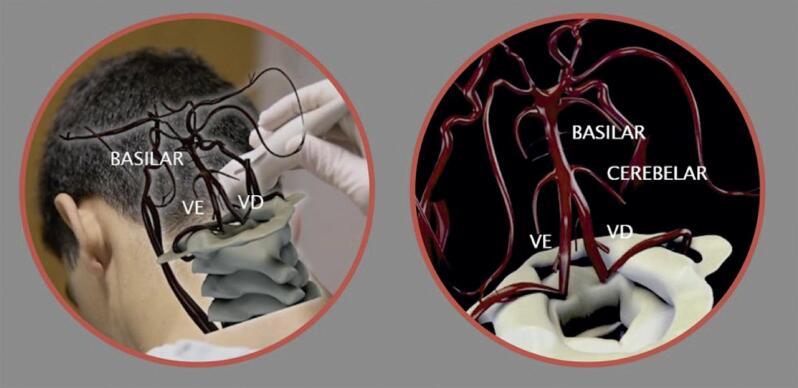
Doppler transcraniano e janela foraminal (12A); representação esquemática dos de vertebrais e basilar (12B). VD: vertebral direita; VE: vertebral esquerda.O paciente deve ser posicionado em decúbito lateral, com queixo tocando o tórax para exposição da região occipital (topografia do forame magno), poderá ainda ser colocado sentado no leito ou em uma cadeira, facilitando o posicionamento do examinador. O Doppler pulsátil evidenciará o fluxo afastando-se do transdutor em luzes de vertebrais e basilar; e nas cerebelares posteroinferiores, a direção será inversa.
